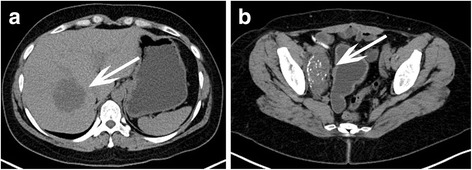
Abdominal and pelvic CT scan at the time of disease recurrence. a Liver metastatic focus (*white arrow*). b Local recurrence in the region of the right iliac vessels (*white arrow*)
